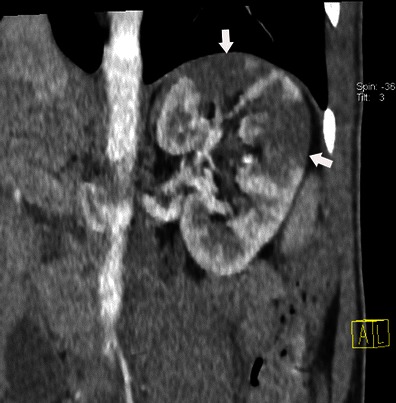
Abdominal contrast-enhanced CT shows multiple hypodense triangular areas on the left kidney. These lesions have a triangular shape with their broadest base on the periphery of the kidneys (*white arrows*)
